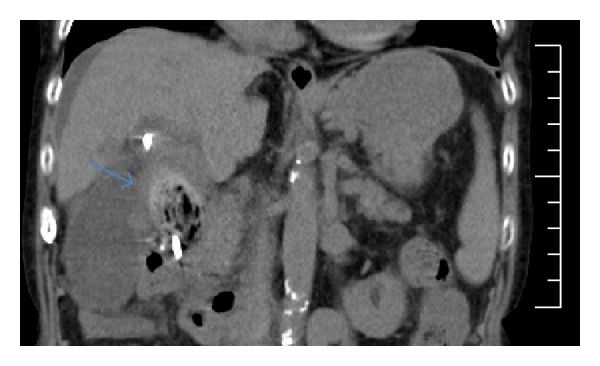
Abdominal computed tomographic (CT) image showing the common bile duct stone (arrow) accompanied by fat stranding in the surrounding adipose tissue on hospital day 47.
